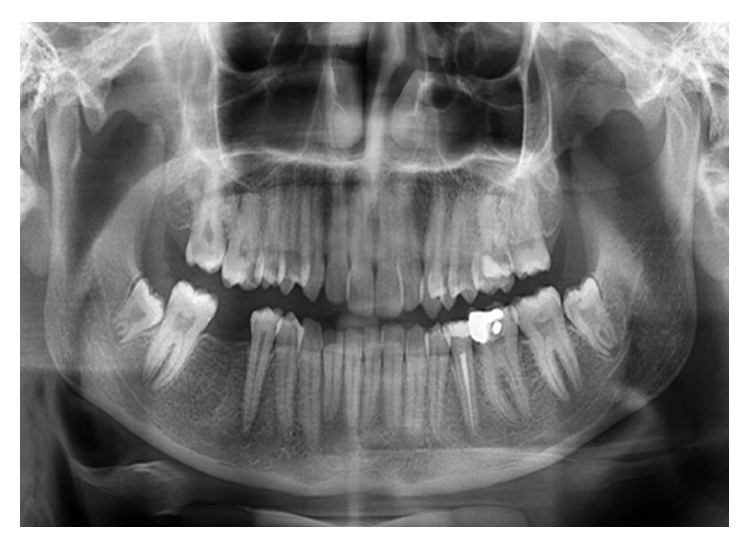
Follow-up panoramic radiograph taken after 1 year. Periapical lesion of mandibular second premolar is completely disappeared.
